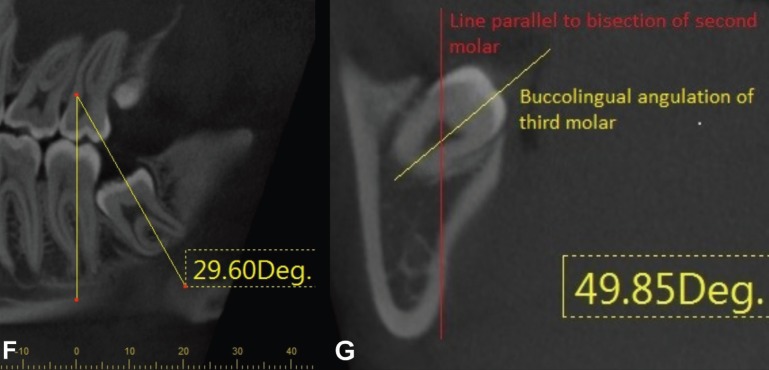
F-Mesio-distal angulation of the impacted third molar. G- Bucco-lingual angulation of the impacted third molar on an coronal CBCT slice.
